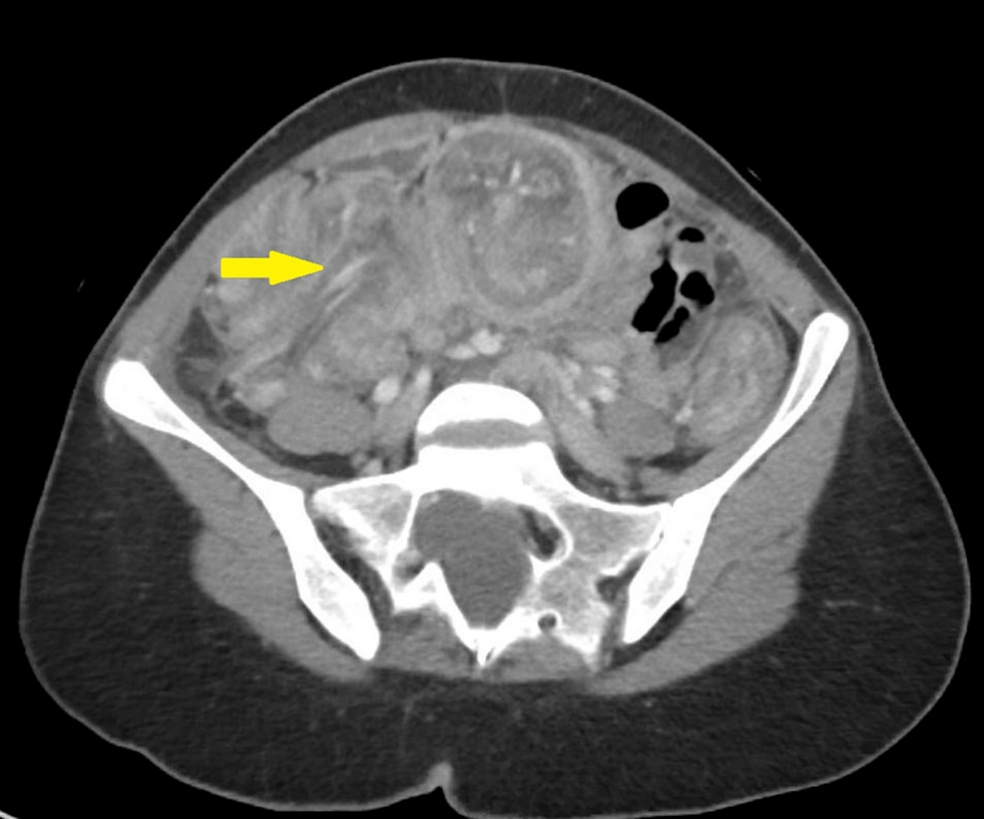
Cecal-colonic intussusception in the axial cut of abdominal computed tomography scan (yellow arrow)
